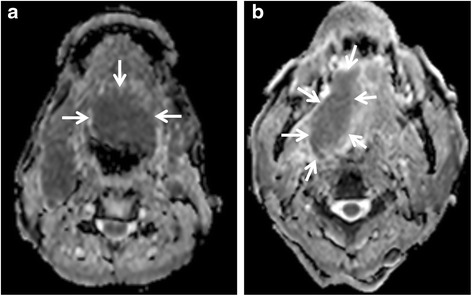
a & b. Pre-treatment DWI for prediction of CRT response. a Pre-treatment ADC map of a squamous cell carcinoma of the tongue (*arrows*) with a low ADC (0.73 × 10^−3^ mm^2^/s) in a tumour with local control; b Pre-treatment ADC map of a squamous cell carcinoma of the tongue (arrows) with a high ADC (1.10 × 10^−3^ mm^2^/s) in a tumour with local failure
